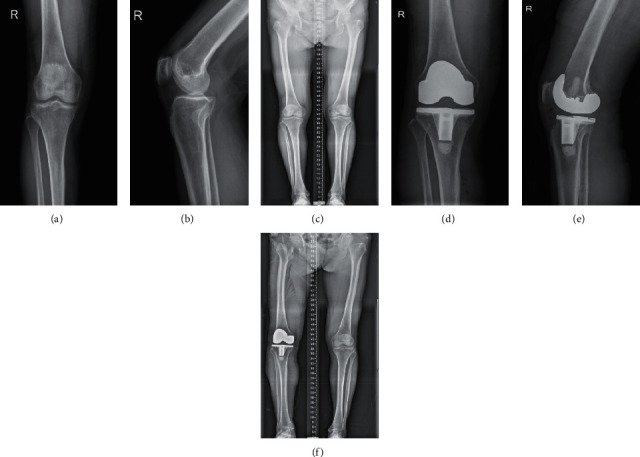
Patient, female, 67 years old. The pain in the right knee has been aggravated for more than 10 years. The osteoarthritis of the right knee joint was treated with total knee arthroplasty of the right knee. (a, b) The bone composition of the right knee joint is complete, the bone hyperplasia shadow can be seen at the bone edge, the intercondylar eminence hyperplasia, the joint space is under weighed, and there is no obvious abnormality in the surrounding soft tissue. Degeneration of the right knee. (c) Preoperative X-ray of the full length of the lower limb showed that the left knee was obviously valgus deformity and the patella was in the middle. (d, e) Replacement in place, joint space, etc. Changes after right knee arthroplasty. (f) The postoperative X-ray of the whole length of the lower limb showed that the valgus deformity of the knee joint was corrected, the prosthesis was in a good position, and the force line of the lower limb was restored.
